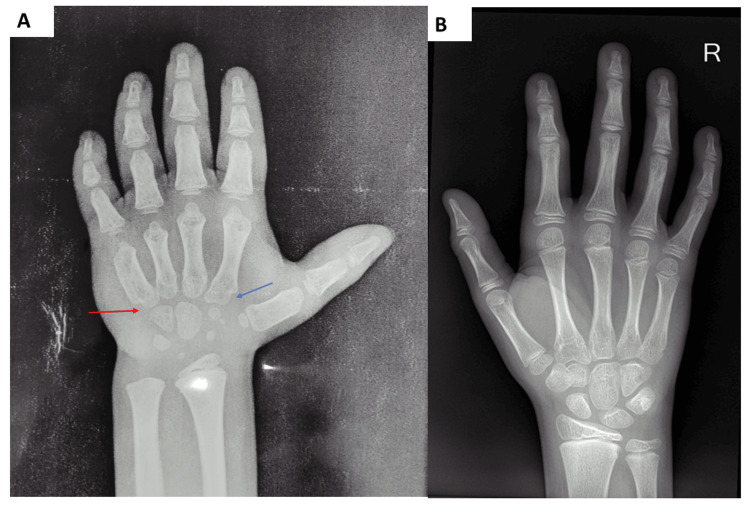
X-ray of a hand with wrist anteroposterior view of the index case (Figure 3A) and the hand of a healthy child of the same age (Figure 3B) The X-ray of the index case (Figure 3A) showed short bones in the hand; conical epiphyses of the phalanges; small round upper epiphyses of the metacarpals; internal notching of the second metacarpal (Figure 3A, blue arrow); and external notching of the fifth metacarpal (Figure 3A, red arrow).
